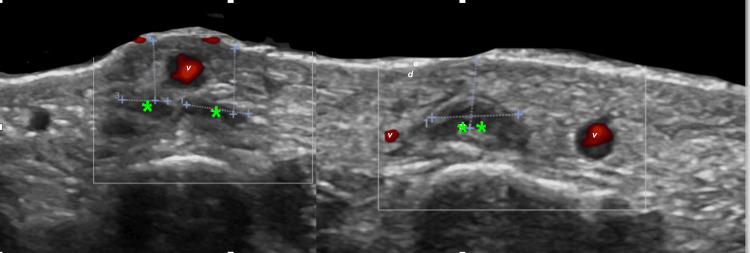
Sonographic appearance after combined PLLA and HA injection (transverse view) (A, B) Green asterisks (*) mark pseudocystic, hypoechoic HA deposits. Horizontal and vertical purple calipers (+) show transverse diameter and depth from the epidermal surface, respectively. Epidermis (e), dermis (d), and the superficial venous plexus (v) are labeled. Abbreviations: PLLA, poly-L-lactic acid; HA, hyaluronic acid
